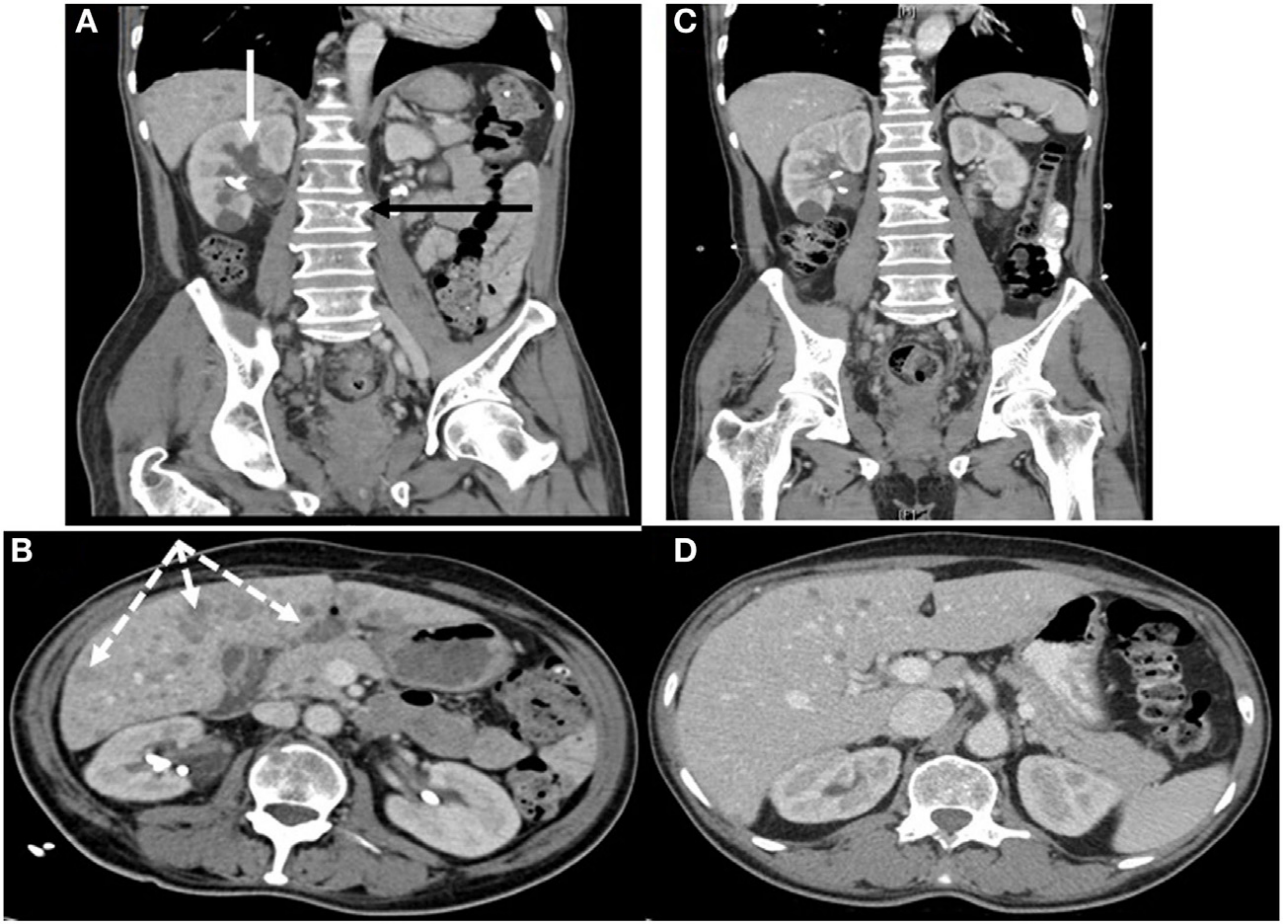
Contrast-enhanced CT scans of chest, abdomen, and pelvis (A,B) showing renal, hepatic, and osseous metastases before chemotherapy (C,D) 2 weeks afterward showing significant interval improvement. (A) Hypoattenuated masses in the right renal pelvis and ureter (solid white arrow) and lytic lesions in the spine (black arrow). (B) Multiple hypoattenuated hepatic masses (dashed white arrows).
